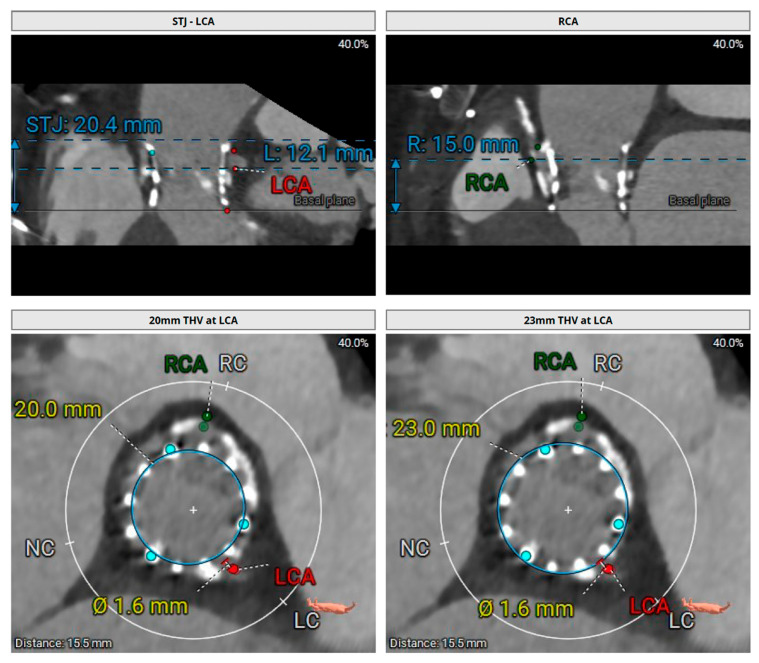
An Unfavorable Case for Re-do TAVR. Pre-procedure CT scan of TAVR valve deterioration showing high-risk anatomy noted by the sinus sequestration which occurs with small valve-to aorta clearance at the STJ (<2 mm) which can jeopardize re-access. Example of TAVR CT images from authors’ institution.
